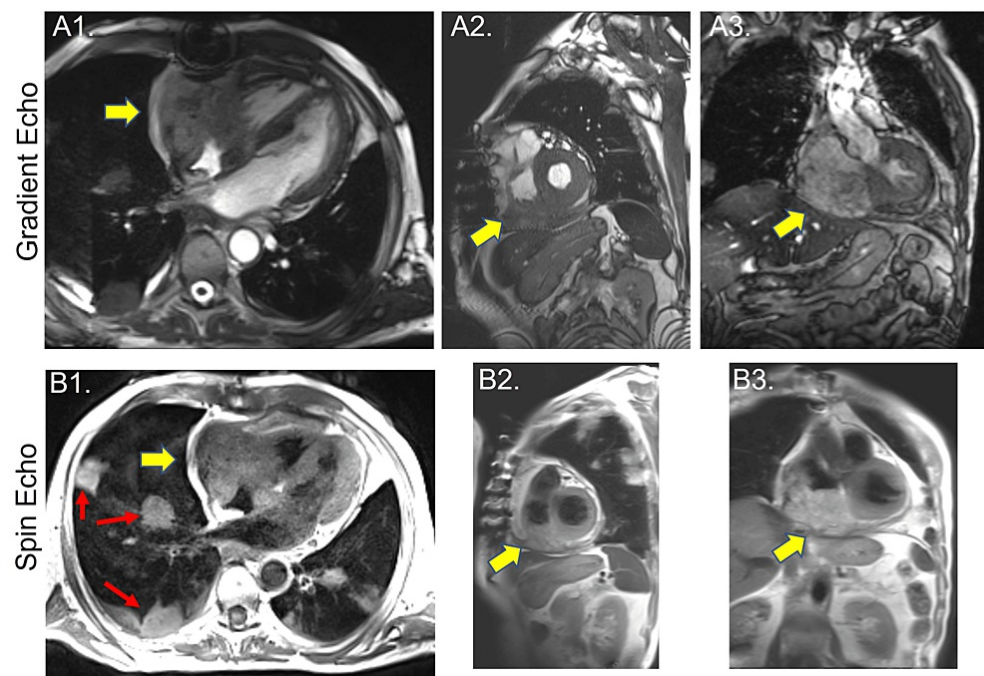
Cardiac magnetic resonance images of cardiac sarcoma Top panels show steady-state free precession gradient echo images, and bottom panels show spin echo images of a large mass in the right atrium extending to the tricuspid valve apparatus and right ventricular inflow (yellow arrows; A1 and B1 four-chamber view, A2 and B2 short axis view, A3 and B3 coronal view). Red arrows show lung masses.
